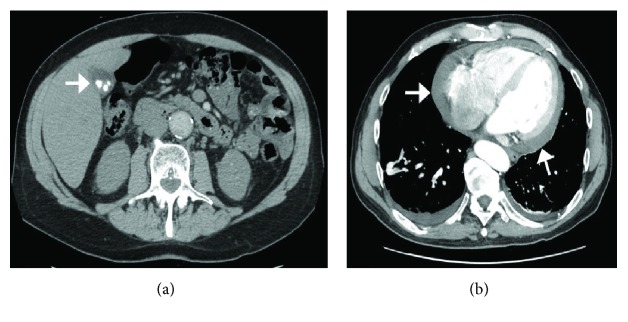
CT scan of the abdomen. (a) Showing gallstones (arrow) with questionable gallbladder wall thickening or pericholecystic fluid. (b) Moderate amount of fluid in the pericardial space appears to be of increased density compared to simple fluid, suggestive of hemorrhage (arrow).
